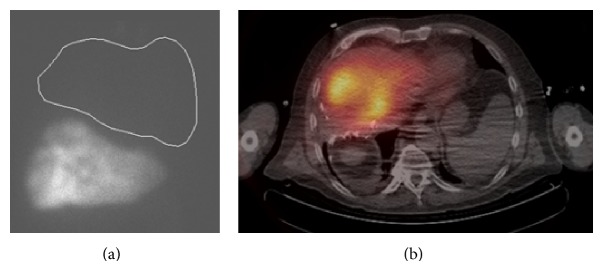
(a) Tc-99 MAA scintigraphy after pre-SIRT mapping demonstrated 6% lung shunting, within acceptable values (<20%). (b) Post-SIRT Bremsstrahlung SPECT/CT showed heterogeneous tracer distribution throughout the remaining left lobe of the liver. No extrahepatic shunting was seen.
